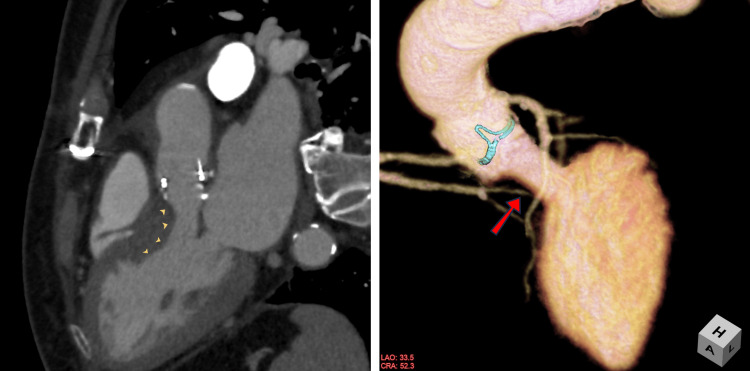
Postoperative CT showed residual ventricular septal muscle (left, arrow head), and narrowed left ventricular outflow tract (right, arrow).
